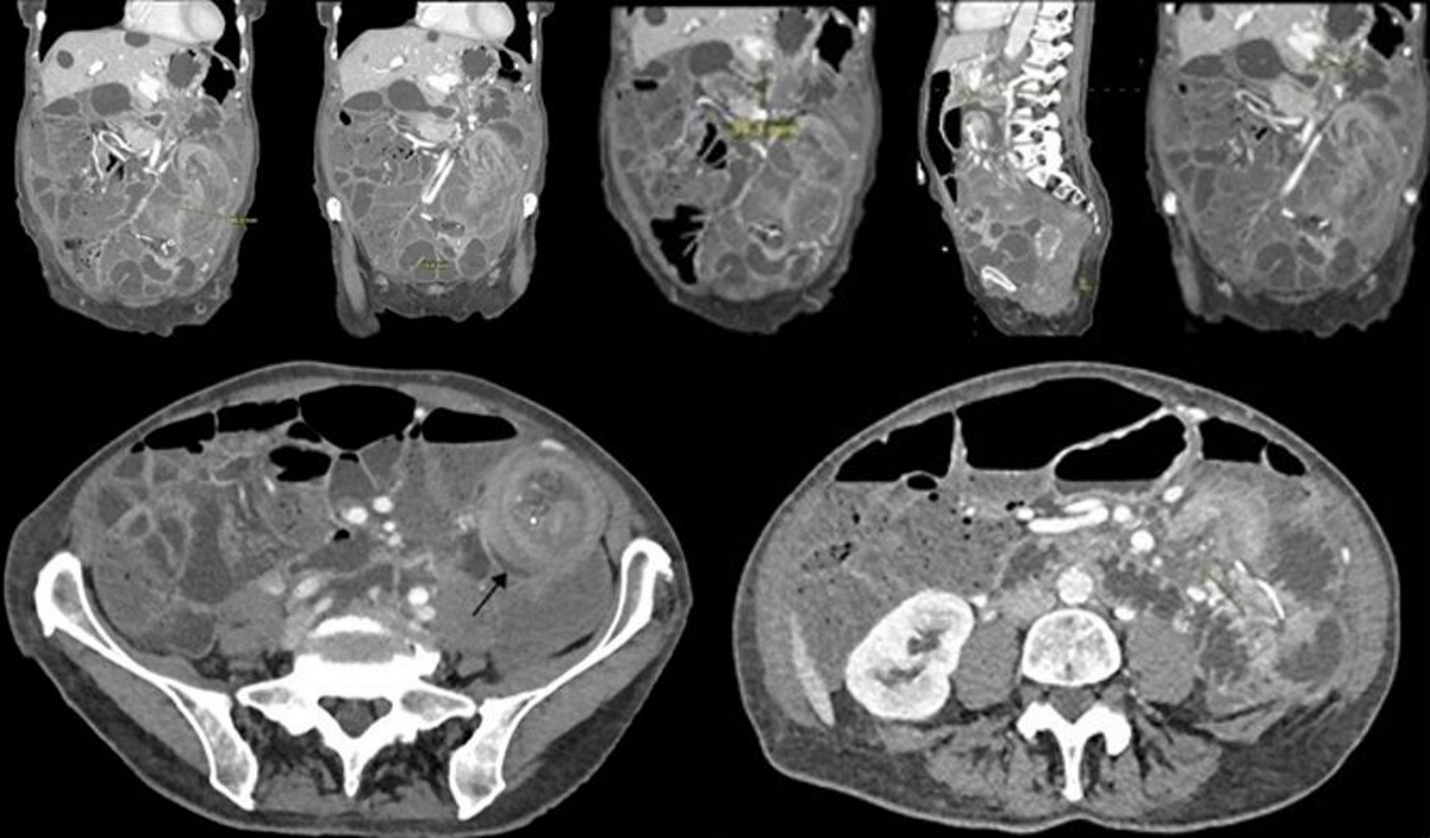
CT scan showing the jejunostomy limb with new long‐segment jejunal–jejunal intussusception (arrow) and associated small bowel obstruction.
